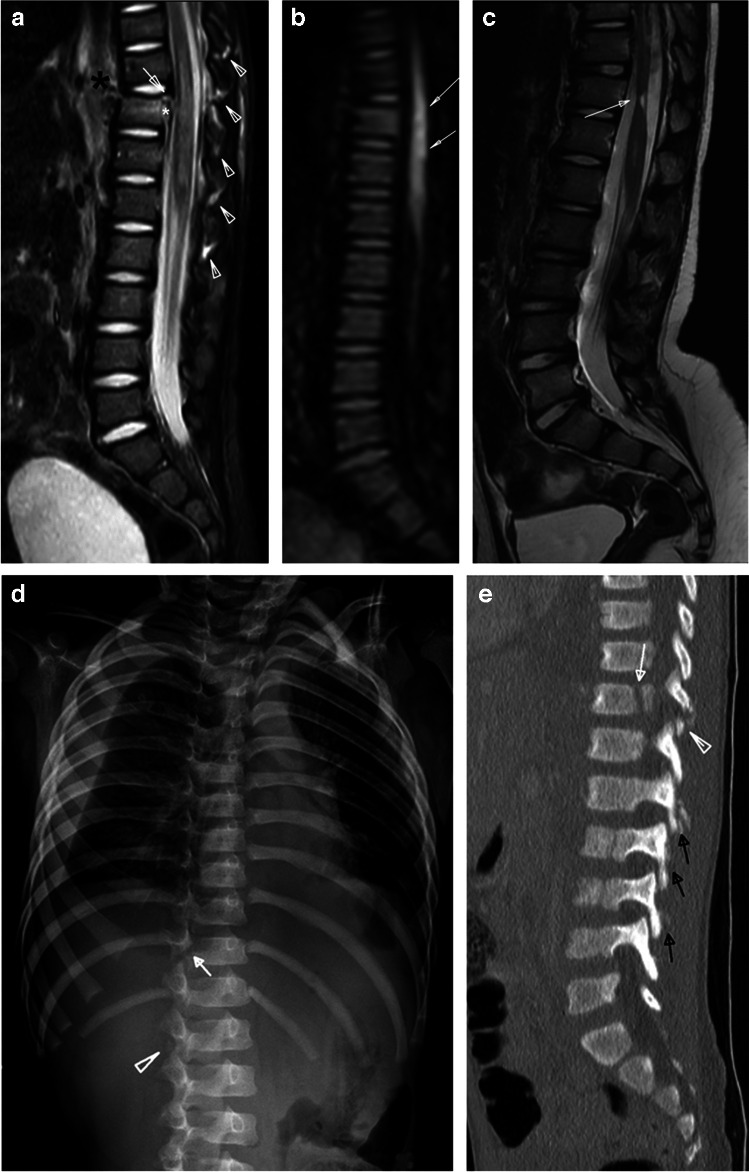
Two-year-old boy with multiple bruises and not moving lower extremities. Sagittal STIR MR image (a) of the lower spine demonstrates fracture of the posterior-superior vertebral body of T11 with disruption of the posterior longitudinal ligament (*arrow*). There is a small volume epidural hemorrhage dorsal to the fracture (*white asterisk*) and large volume retroperitoneal hemorrhage (*black asterisk*) overlying a disrupted anterior longitudinal ligament. Multilevel interspinous ligamentous edema and widening is noted at the thoracolumbar junction (*white arrowheads*). Acute injury to the spinal cord is visible on the sagittal STIR MR image (a) spanning T10-T12 as T2 prolongation and confirmed on a sagittal diffusion image (b) of the cord (*arrows*). A 6-month follow-up sagittal T2-weighted MR image (c) of the cord demonstrates sequelae of the cord injury with myelomalacia (*arrow*) at site of the previous injury. Oblique radiograph of the ribs (d) demonstrates subtle lucency through the right T11 neurocentral synchondrosis (*arrow*) and heterotopic bone formation adjacent to the right L1 facet joint (*arrowhead*), which were overlooked on the initial skeletal survey, but correspond with the interspinous edema and widening seen on prior MR (a) and fractures better seen by CT. Sagittal bone algorithm reconstruction from an abdominal CT with contrast (e) demonstrates the neurocentral synchondrosis fracture (*arrow*), facet injury (*arrowhead*), and heterotopic bone formation at multiple levels of the lumbar spine (*black arrows*)
